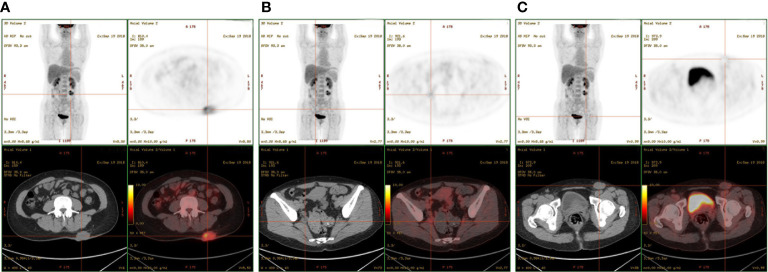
PET-CT showed multiple masses located in the (A) left lower back, (B) right pelvic wall, and (C) inguinal region.
